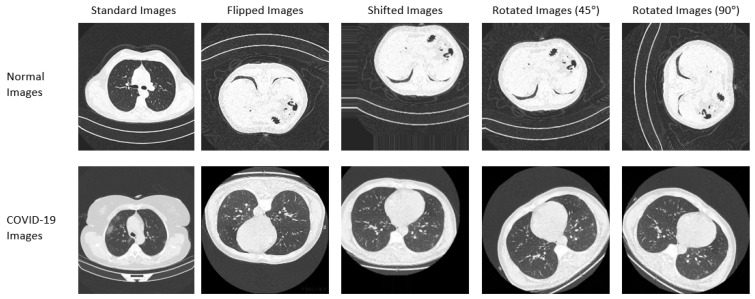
Samples of standard and augmented CT images used for training.
